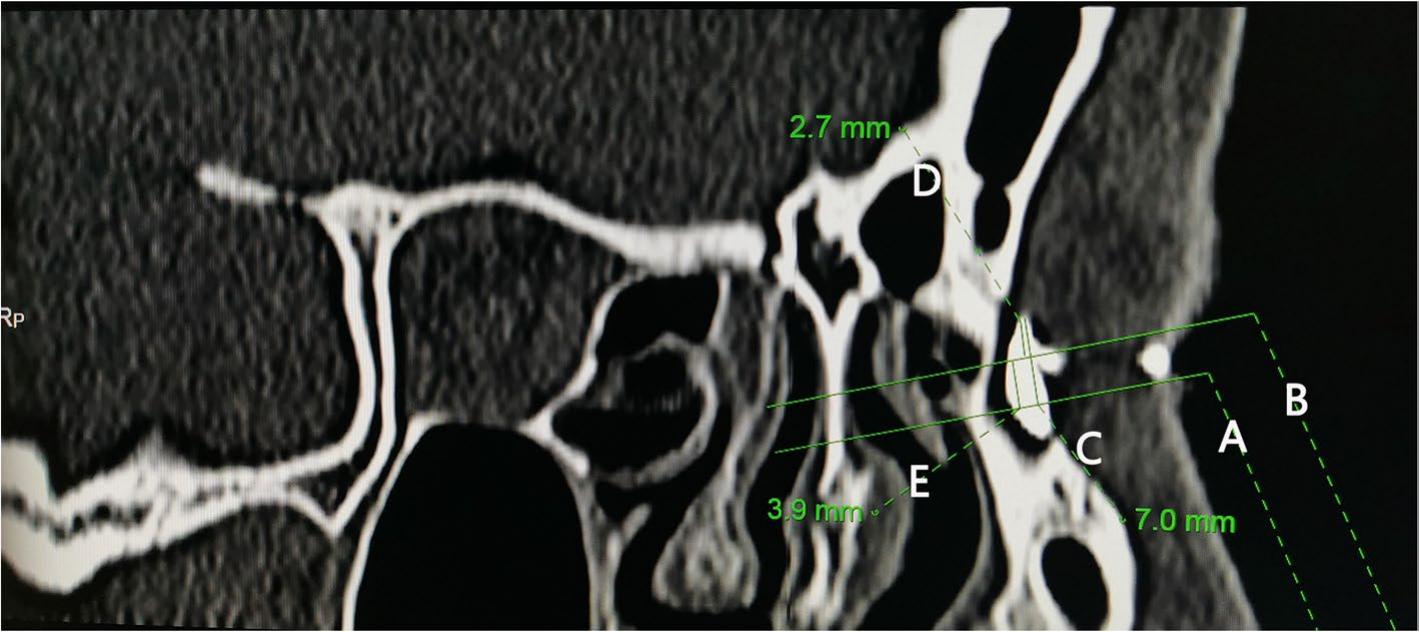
Measurements in the long axis of a medium lacrimal sac on computed tomographic dacryocystography (CT-DCG) parasagittal images. A, a line drew from the middle turbinate axilla (MTA) to the long axis of the sac at right angles. B, a line drawn through the common canaliculus (CC) to the long axis of the sac at right angles. C, the height of the sac above the MTA. D, the height of the sac above the CC. E, the distance from the MTA to the CC
